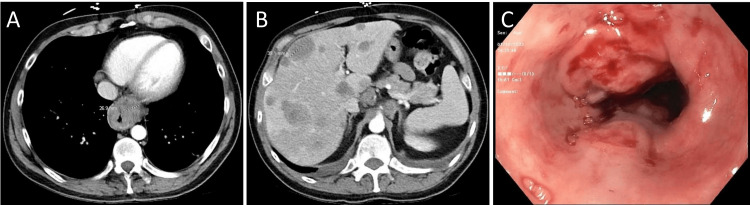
Case 1 images A: CT showing an esophageal heterogeneous mass that is 26.9 mm thick in the distal third of the esophagus. B: CT showing innumerable solid hypodense hepatic masses (largest measuring 39.1 mm) with associated upper abdominal lymphadenopathy. C: EGD showing a large, non-obstructing, circumferential, ulcerating mass in the lower third of the esophagus. EGD: esophagogastroduodenoscopy
